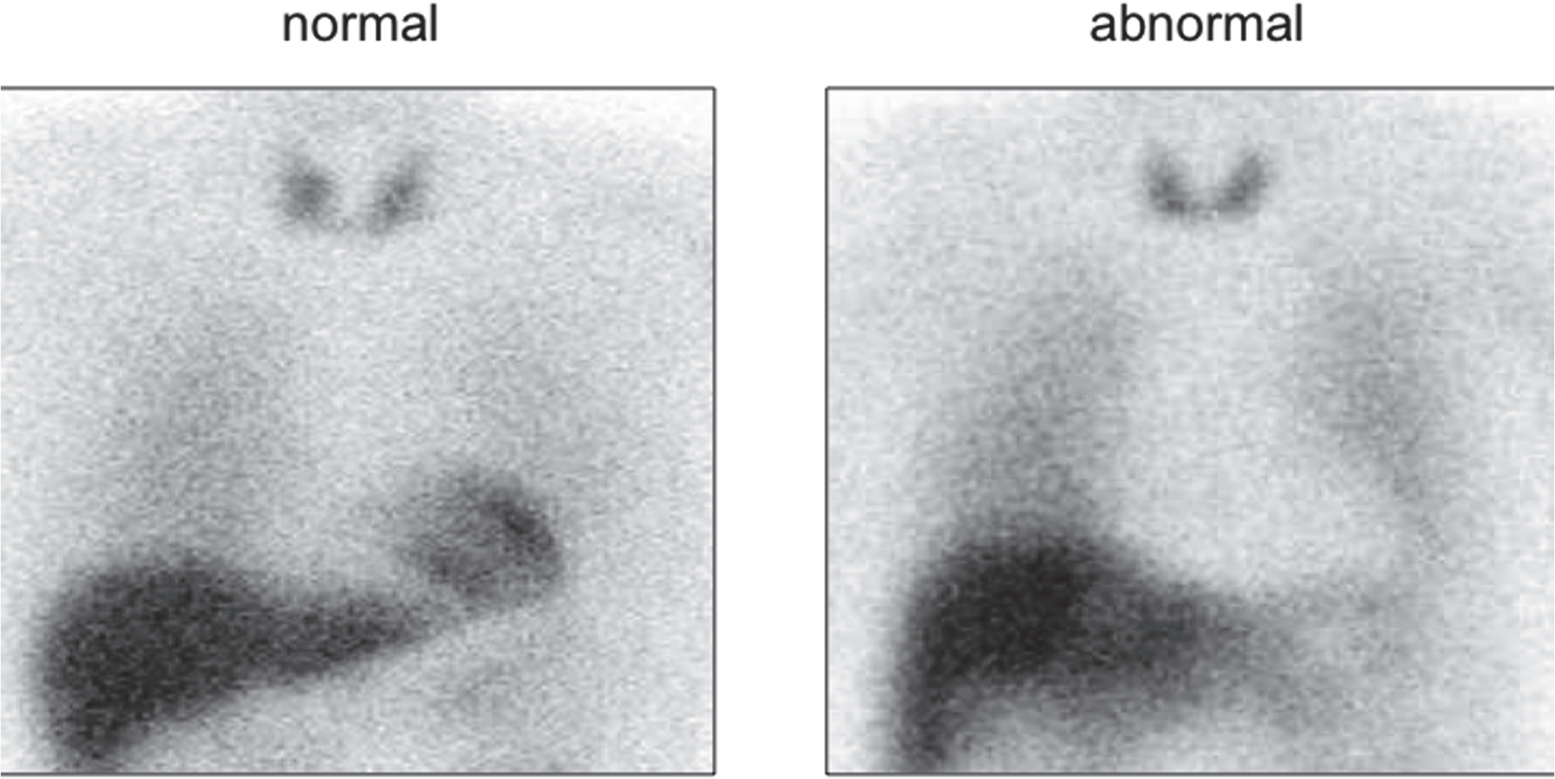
Normal and abnormal planar image of ^123^I-MIBG cardiac scintigraphy.
